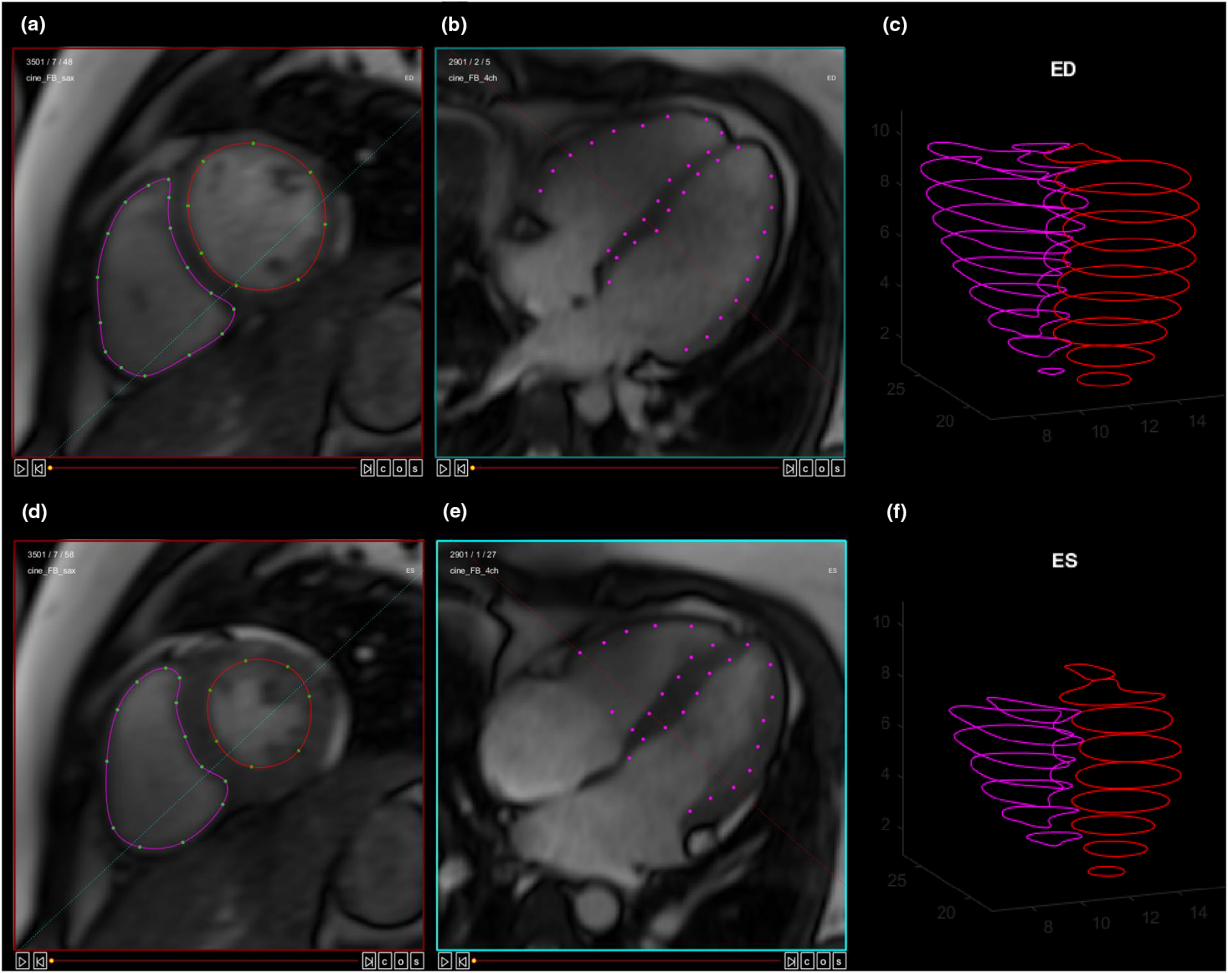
Example of real‐time ungated free‐breathing cardiac MRI imaging. Endocardial borders of the left (red contours) and right ventricle (pink contours) were traced on short‐axis images at (a) end‐diastole (ED) and (d) end‐systole (ES). Transection of the short‐axis contours with the horizontal long‐axis plane for the corresponding phase of the cardiac cycle (b, e) is shown by the pink dots. Areas for each short‐axis slice at ED (c) ED and (f) ES are summed using the summation of discs method to determine ED and ES ventricular volumes.
